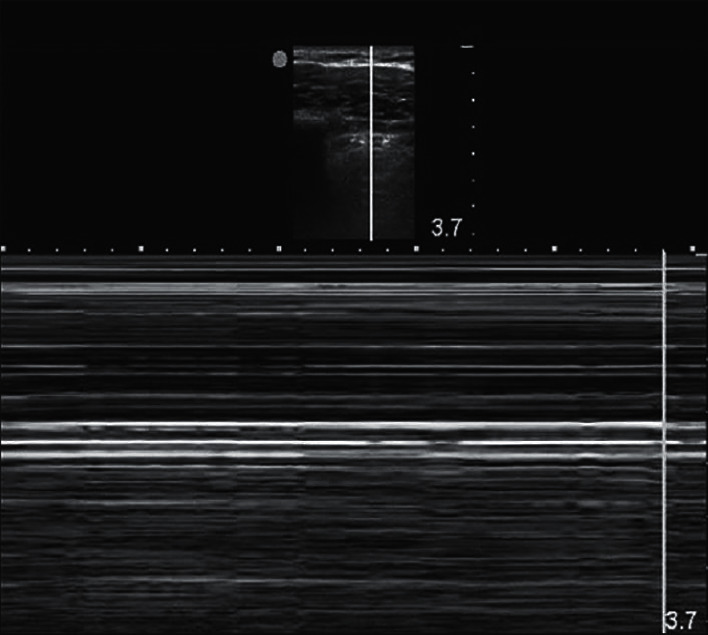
Transthoracic ultrasound showing “barcode” in M-mode from [Abdalla W., Elgendy M., Abdelaziz A. A., Ammar M. A. Lung ultrasound versus chest radiography for the diagnosis of pneumothorax in critically ill patients: a prospective, single-blind study. Saudi Journal of Anaesthesia 2016; 10 (3): 265–269].
